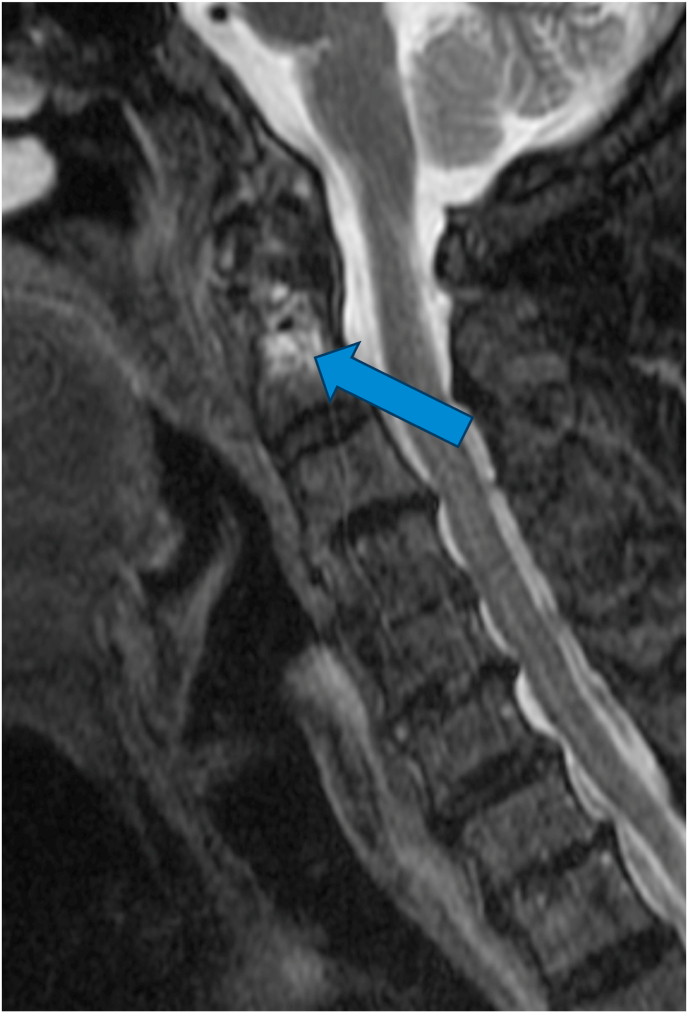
MRI showing edema in the dens (straight arrow).
